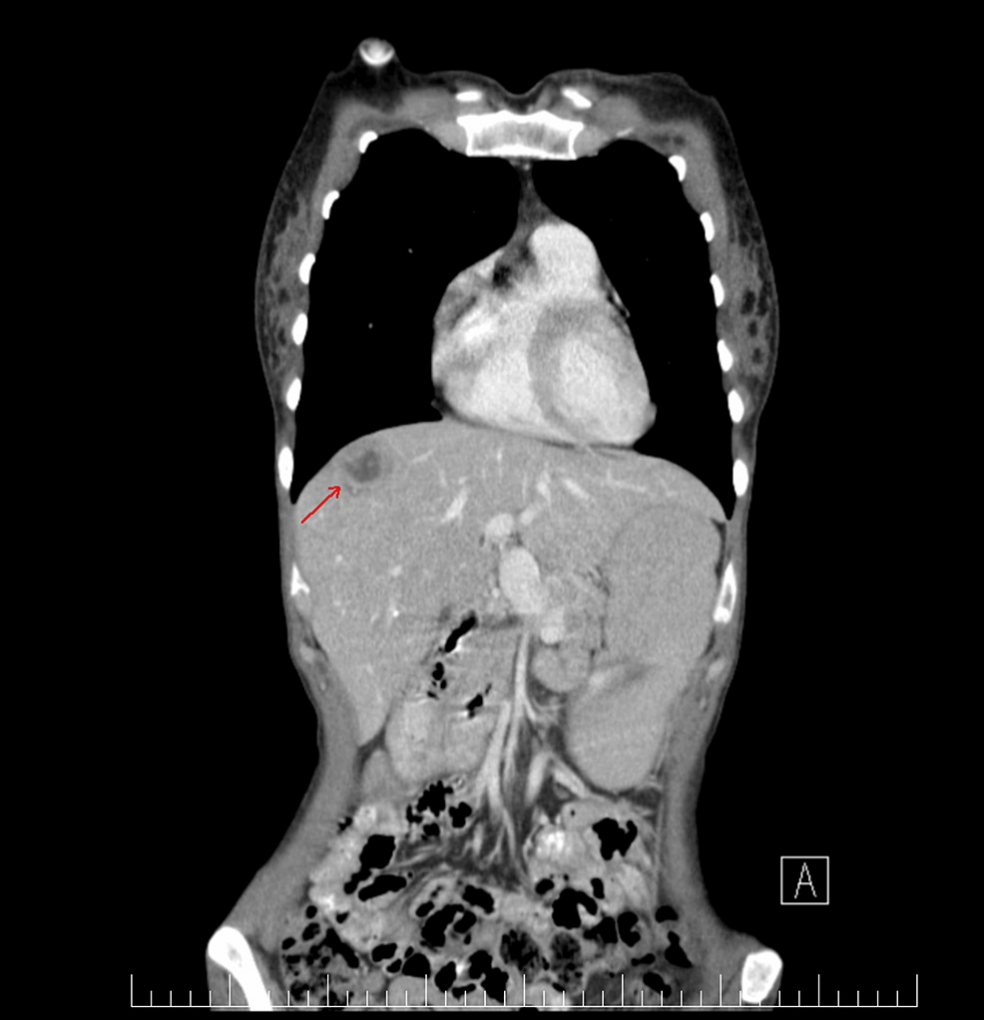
Coronal view of thoraco-abdomino-pelvic CT scan. The liver has normal dimensions and regular contours. Within the VIII segment, there is a hypodense lesion that is well-delimited, with axial diameters of approximately 51 mm in anteroposterior diameter and 45 mm in laterolateral diameter. This lesion exhibits spontaneous iso/hyposignal and is non-encapsulated. During the arterial phase, there is early enhancement, except for a fibrotic central area, and a tendency toward homogenization is observed in the late sequences. Additionally, areas of hypervascularization are noted.
